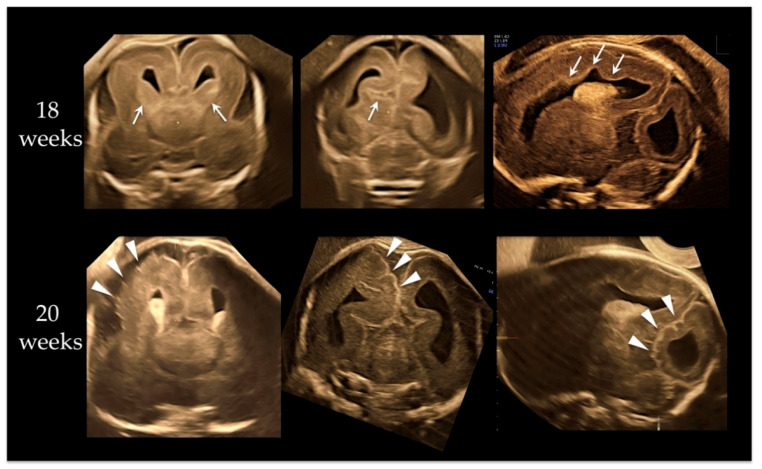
Neurosonographic findings at 18 and 20 weeks of gestation. Upper three images are at 18 weeks. From left, anterior coronal, posterior coronal, and parasagittal views. Megalencephaly with asymmetrical structure, broad ganglionic eminence (arrows on upper left image), abnormal sulcation (arrow on upper-middle image) on cerebral cortex, and irregular ventricular wall (arrows on upper right image) were demonstrated. Lower three images are at 20 weeks. From left, anterior coronal, posterior coronal, and parasagittal views. A remarkable cortical malformation with multifocal polygyria (arrowheads) was visualized; polygyria were not seen at 18 weeks. Compared to upper images, taken two weeks before, focal cortical dysplasia due to rapid neuronal migration disorder may have occurred randomly in various cerebral cortex regions.
